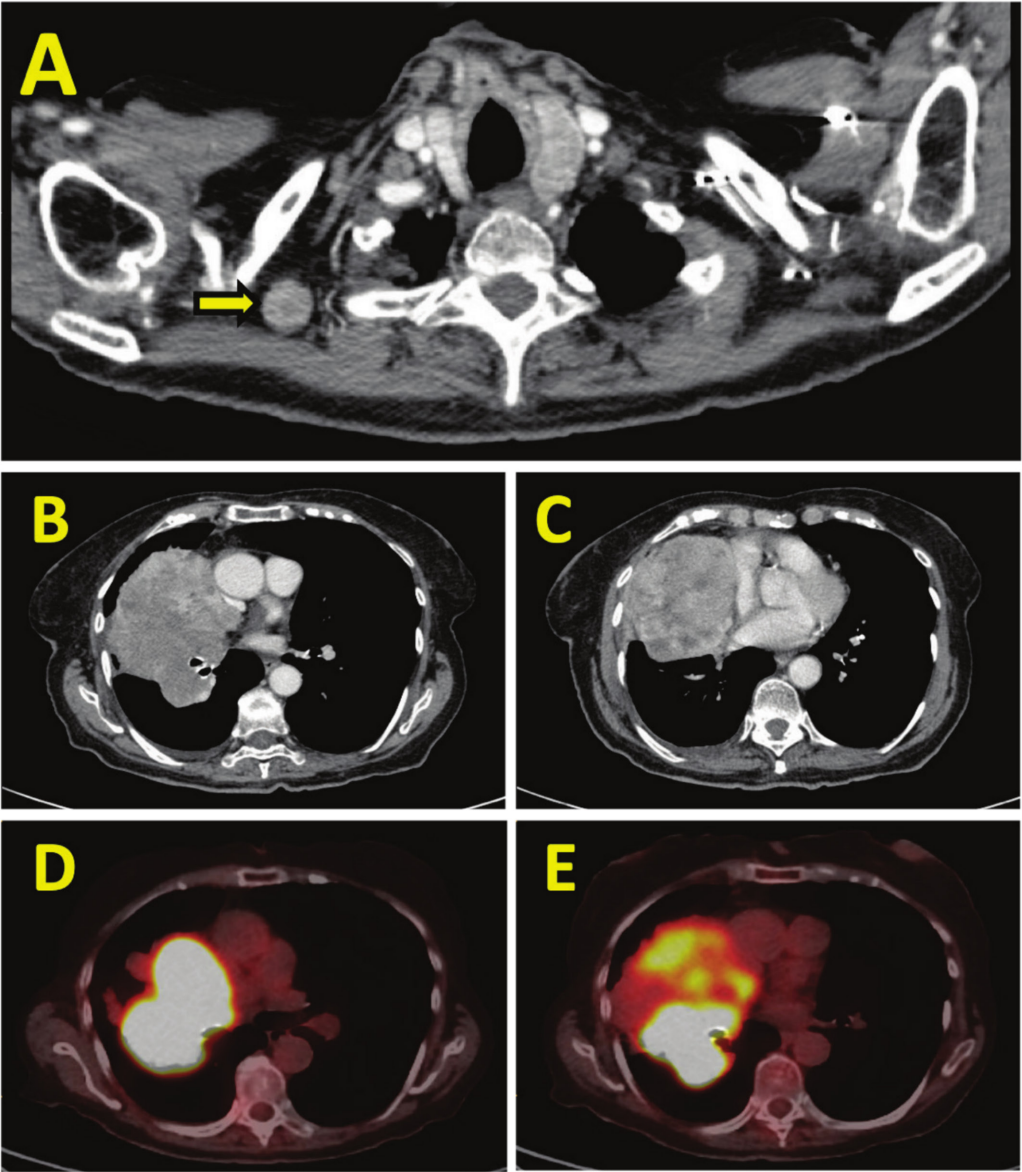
Axial CT chest images: (A) enlarged right supraclavicular lymph node (arrow); (B and C) right lung mass. FDG‐PET scan images: (D and E) demonstrate intense FDG uptake within the lung mass.
